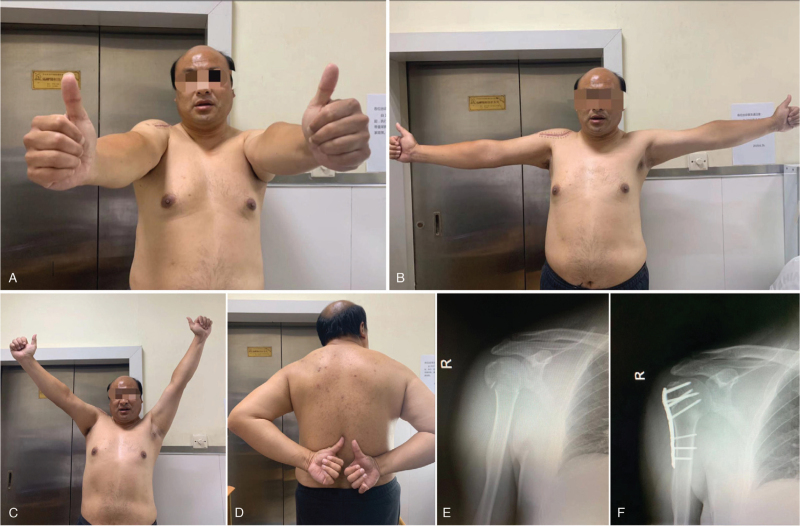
Clinical and functional outcome. (A-D) Function at the 3 mo. (E) Preoperative X-ray. (F) Post-operative X-ray.
